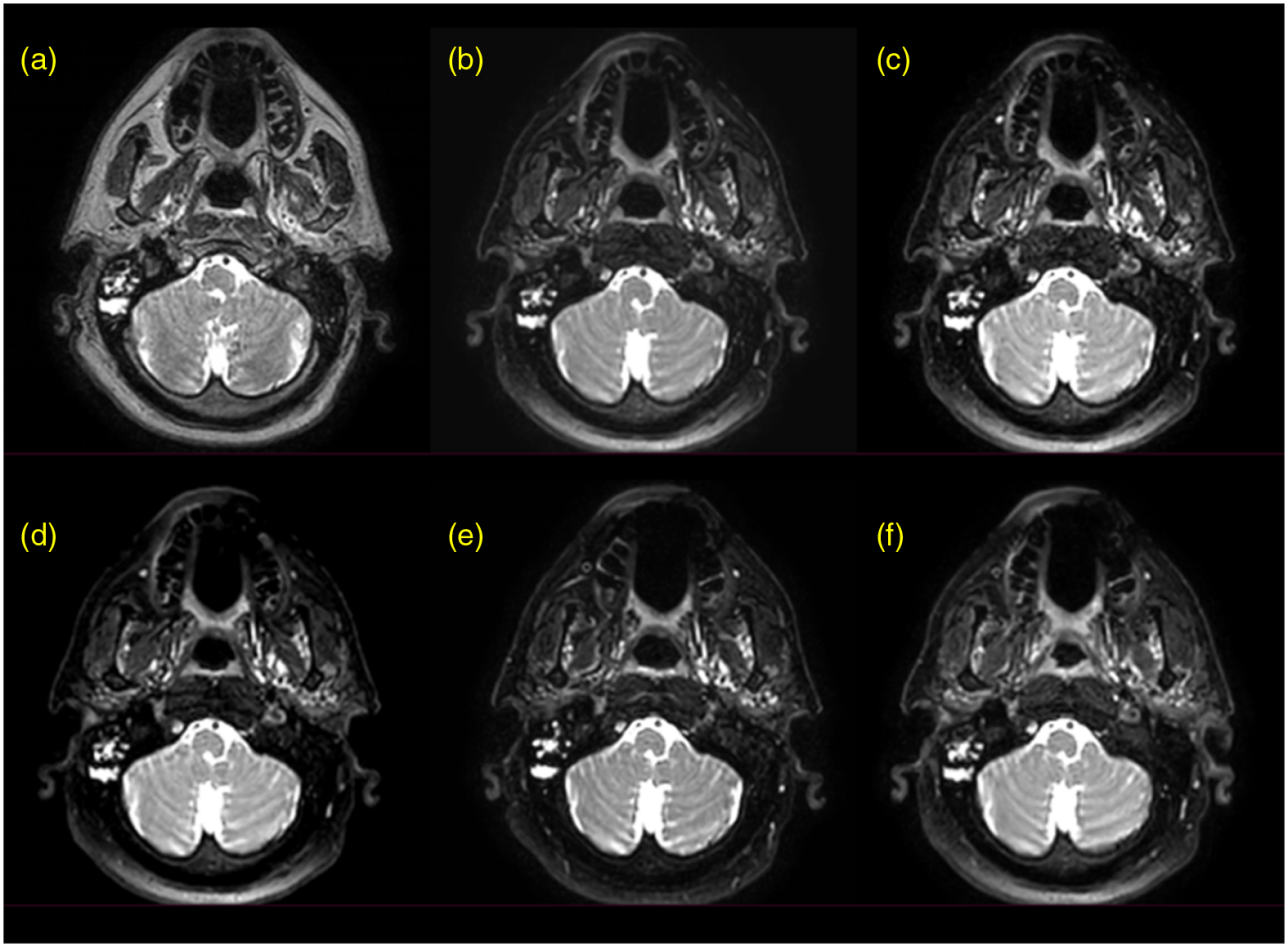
(a) Nonsuppressed, T2-weighted sequence and (b)–(f) five SPAIR sequences acquired on a representative patient with HNC using a Unity magnetic resonance linear accelerator.
